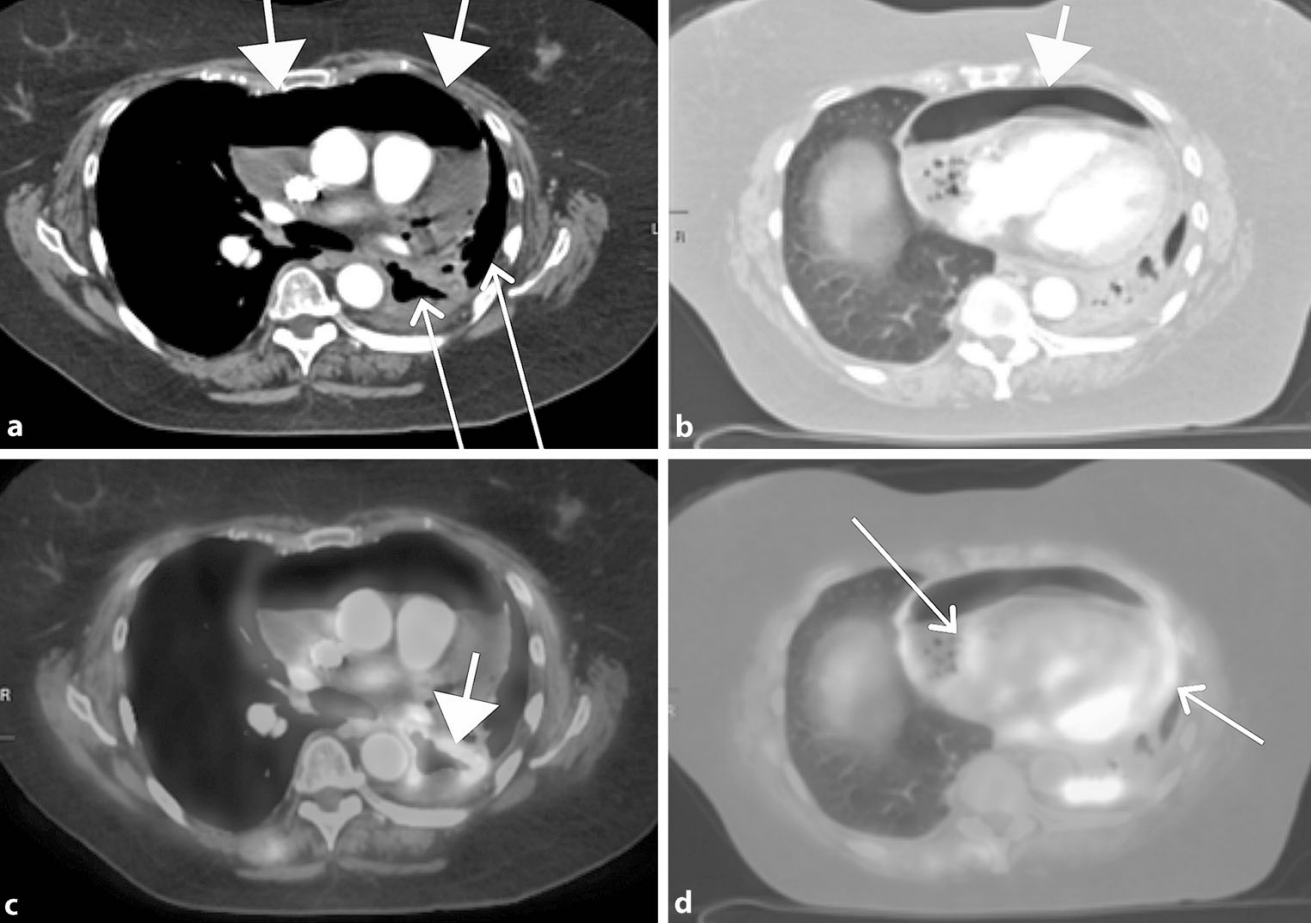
a, b CT demonstrates abnormal communication between the air-containing
